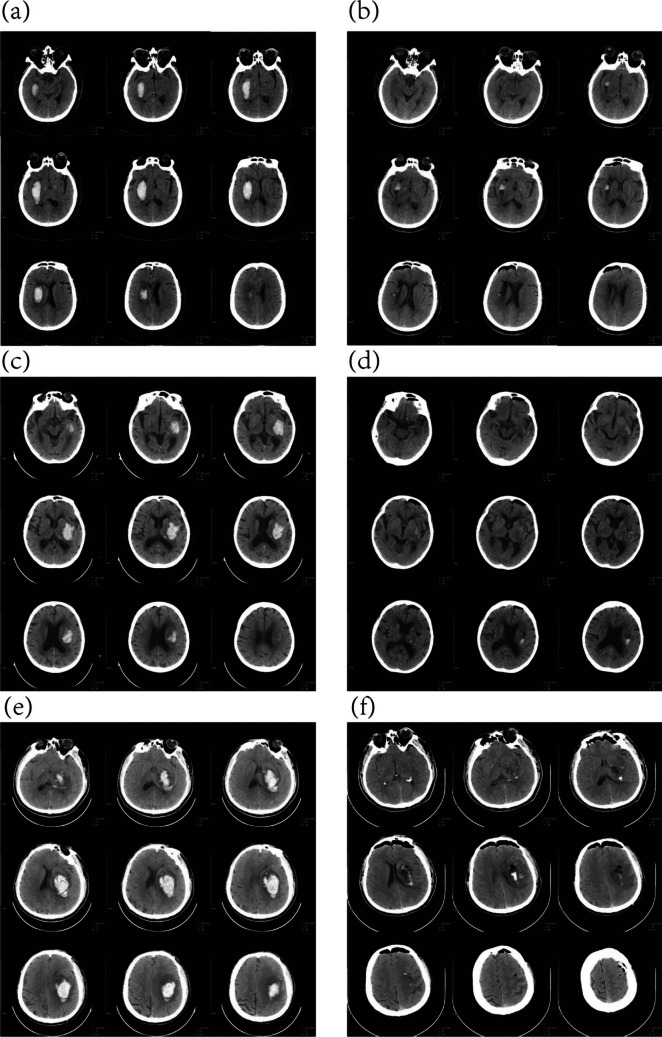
The CT images immediately after ROSA robot-assisted stereotactic removal of intracranial hematoma were compared with CT that before surgery.( First patient:a,b; Second patient:c,d; The third patient:e,f)
